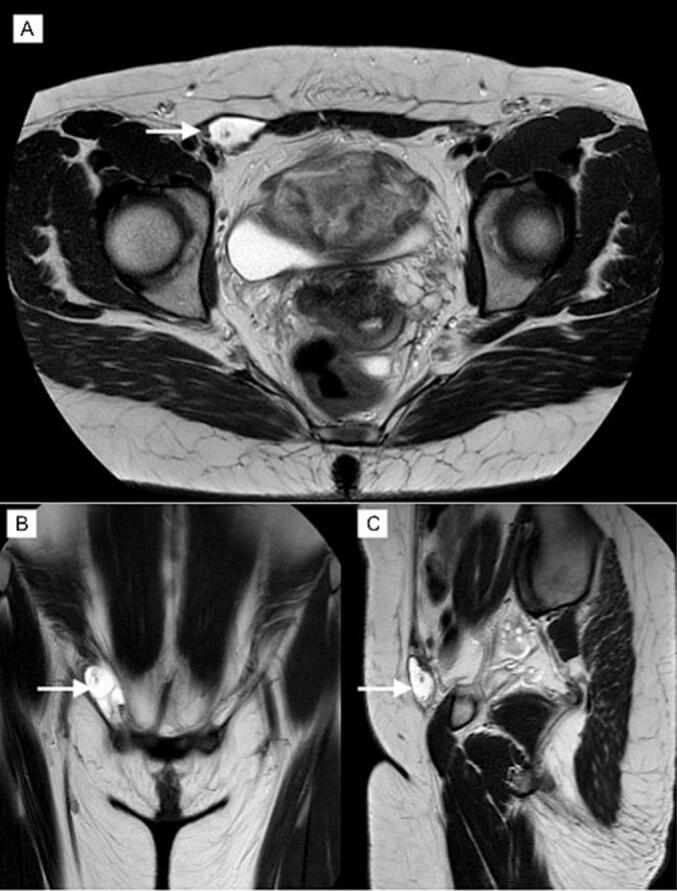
T2-weighted MRI in transverse (A), coronal (B), and sagittal (C). An arrow indicates a 4 × 2.6 × 1.7 cm cystic lesion with thin septations and no communication to the peritoneal cavity.
